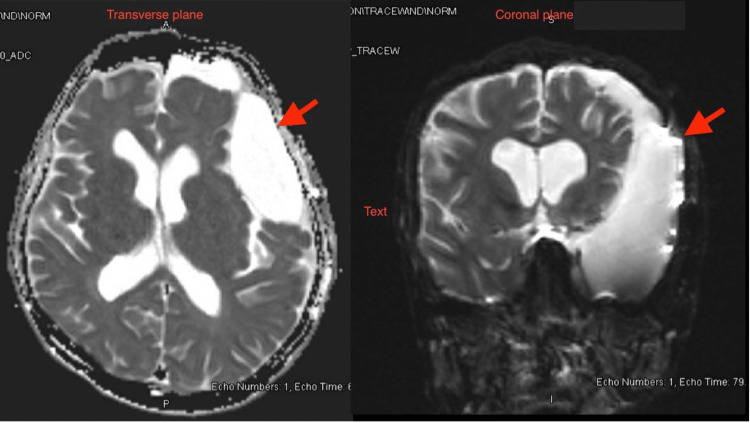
Follow-up CT head scan was done after one week from arachnoid cyst fenestration showing a decrease in the size CT: computed tomography
